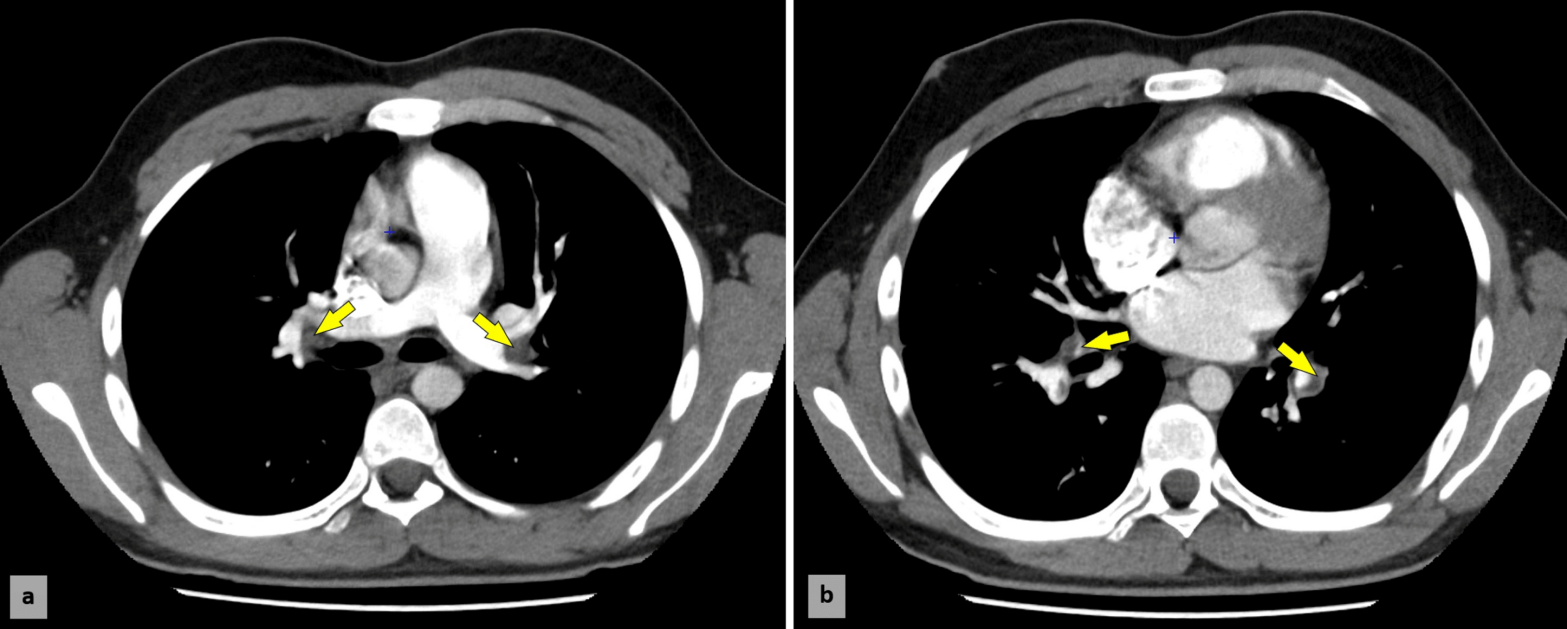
Post-contrast axial CT scan of the thorax reveals bilateral pulmonary emboli (arrows)
